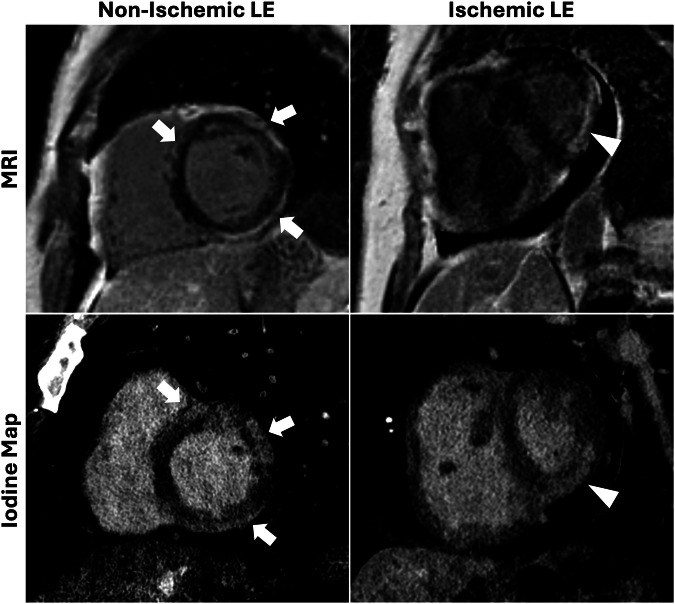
Non-ischemic and ischemic LE case examples. Top row: magnitude short-axis view late gadolinium enhancement images. Bottom row: short axis view of iodine maps generated from PCD-CT. The left column represents a case of a 50-year-old woman with a family history of arrhythmogenic ventricular cardiomyopathy, and subepicardial LE in the left ventricle (arrows), indicating areas of fibrous tissue. The right column is a 72-year-old man with a history of a myocardial infarction and previous stent implantation. A transmural LE is seen on the posterior basal and mid-ventricular wall (arrowhead), as well as a pericardial effusion. LE, late enhancement
